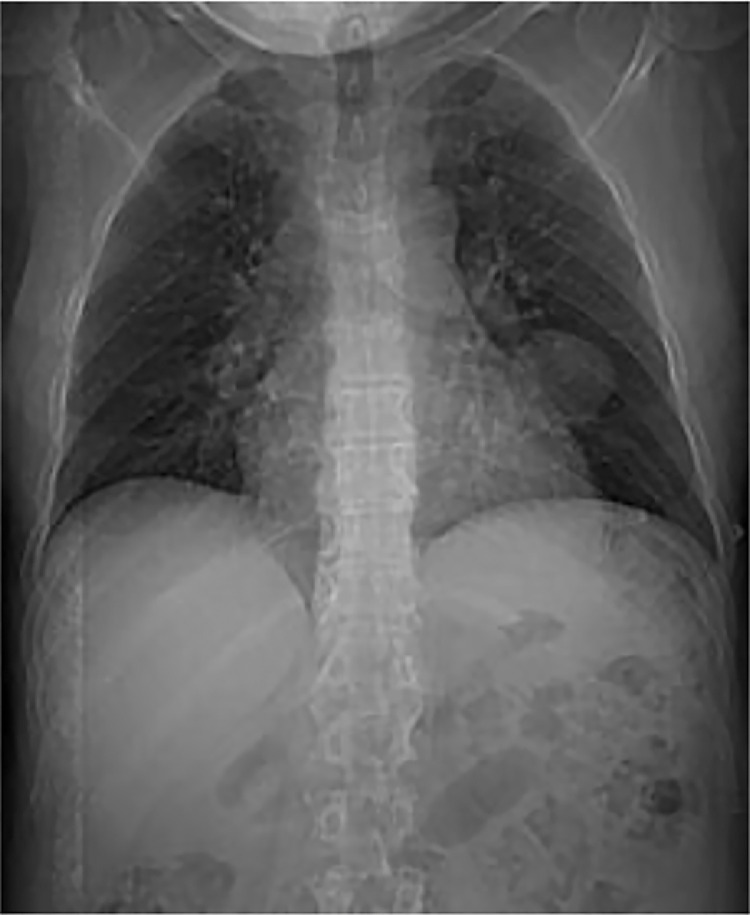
Chest X-ray showed accentuation of the peribroncovasal texture, and reticular thickening. It also showed a large mass lesion on the left.
